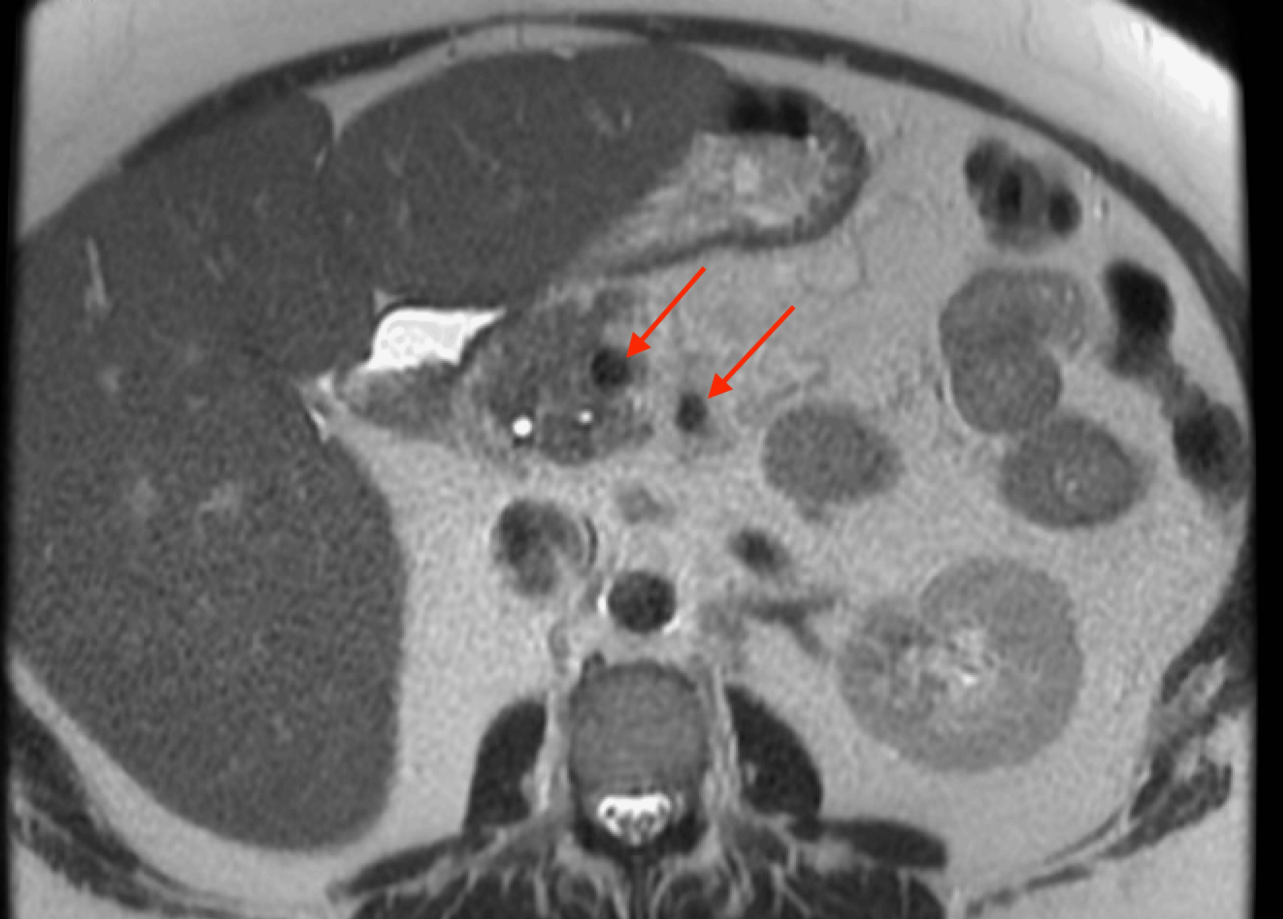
T2-weighted MRI showing peripancreatic lymphadenopathy.
